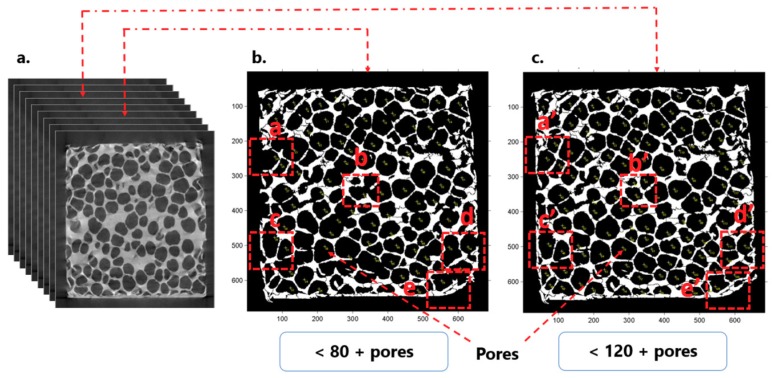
2D tomography images of the closed-cell aluminum foam samples (Aluhab foam): (a) CT scan images; (b,c) random position images, selected from the range of images generated during CT scanning, showing the differences in pore sizes and arrangements, and cell wall thicknesses throughout the 3D volume of the foam sample.
